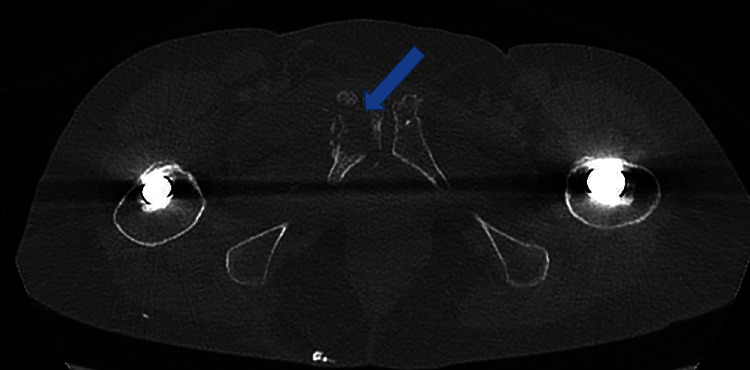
CT scan showing destruction of pubic bone and fluid collection suggesting acute osteomyelitis.
